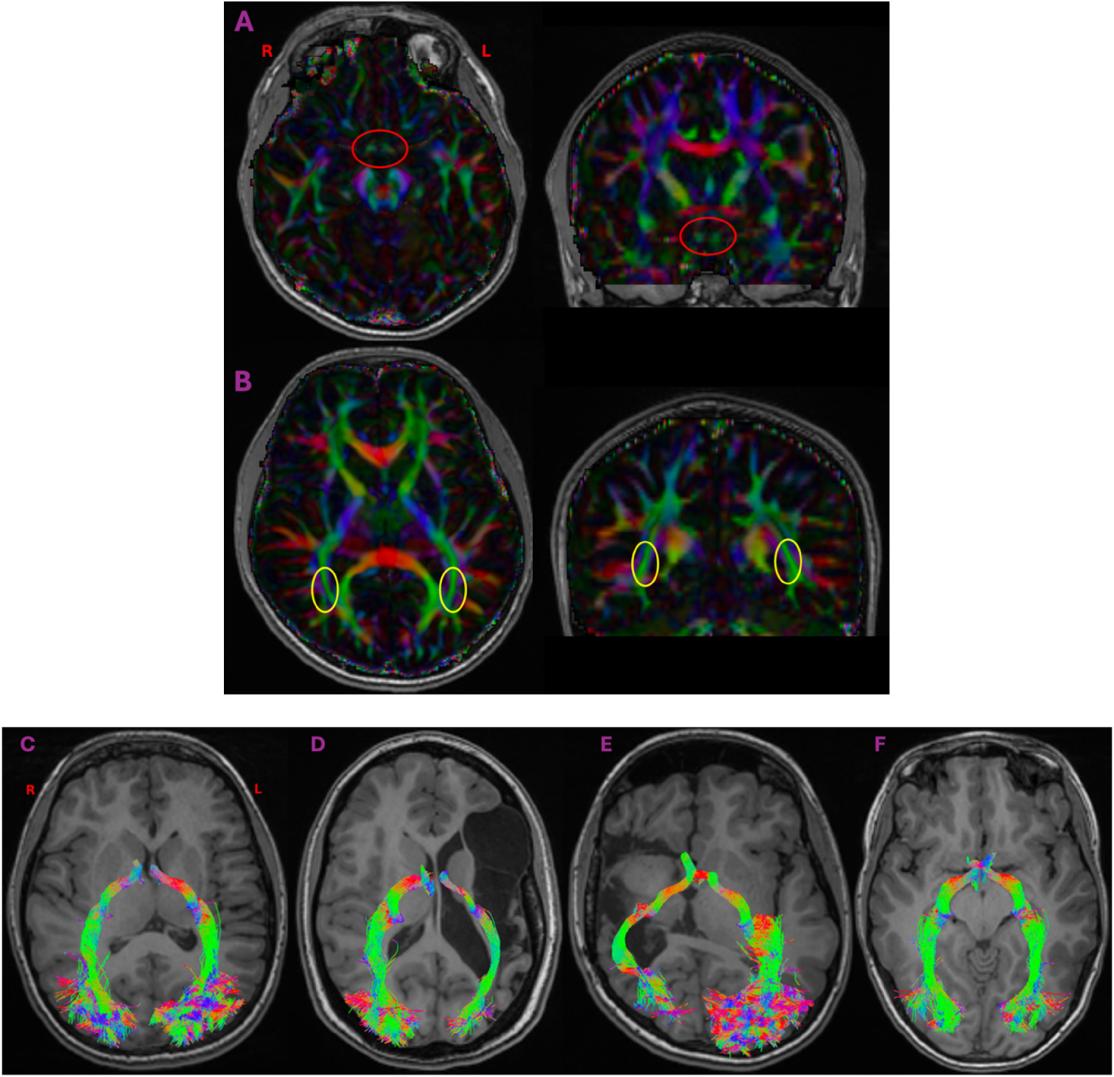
Top: T1-weighted images with a colourmap overlay in axial (left) and coronal (right) views. ROIs located posterior to the optic chiasm (A) and the posterior thalamic radiations (B) were used to generate white matter representations. Bottom: T1-weighted images and reconstructed white matter representations of the optic tracts and optic radiations in 4 participants: 2 with left-hemisphere lesions (C, D), 1 with a right hemisphere lesion (E) and 1 typically developing child (F).
